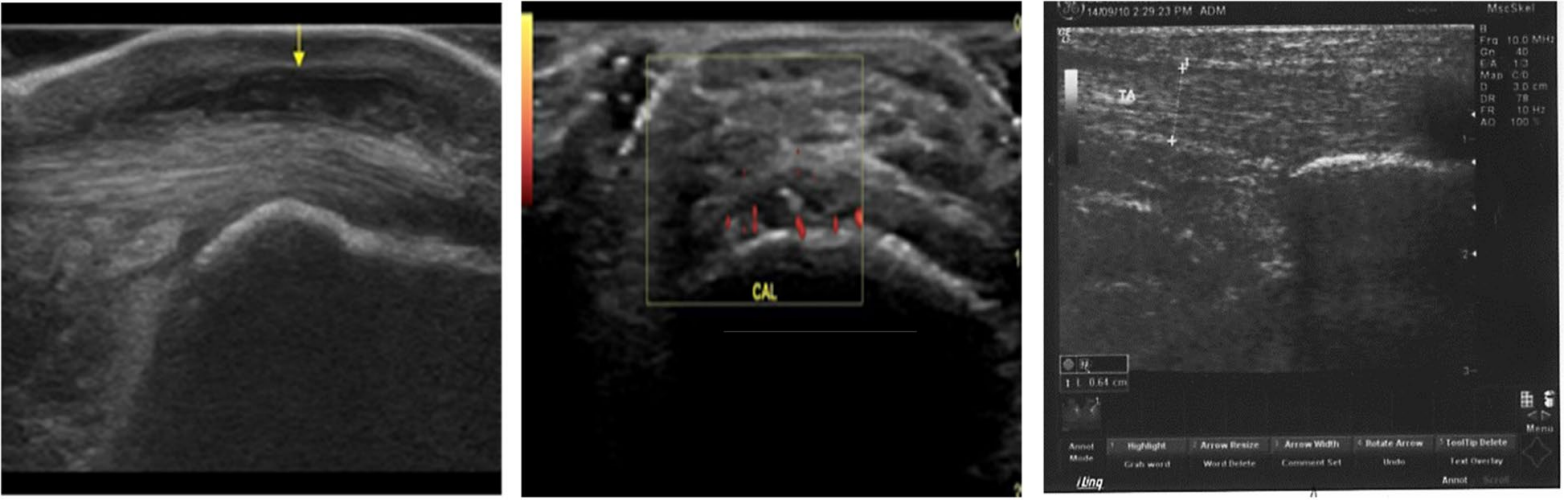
A Longitudinal B-mode superficial ultrasound of the dorsal ankle showing retro calcaneal bursitis seen as hypoechoic thick walled fluid filled bursa at the retro calcaneal space. B Longitudinal color Doppler superficial ultrasound of the planter aspect of the foot showing thickened planter fascia with increased vascularity denoting planter fasciitis, cal (calcaneus(. C Longitudinal B-mode superficial ultrasound at the level of the dorsal ankle showing thickened tendo-Achilles tendon (between calibers 6.4 mm)
